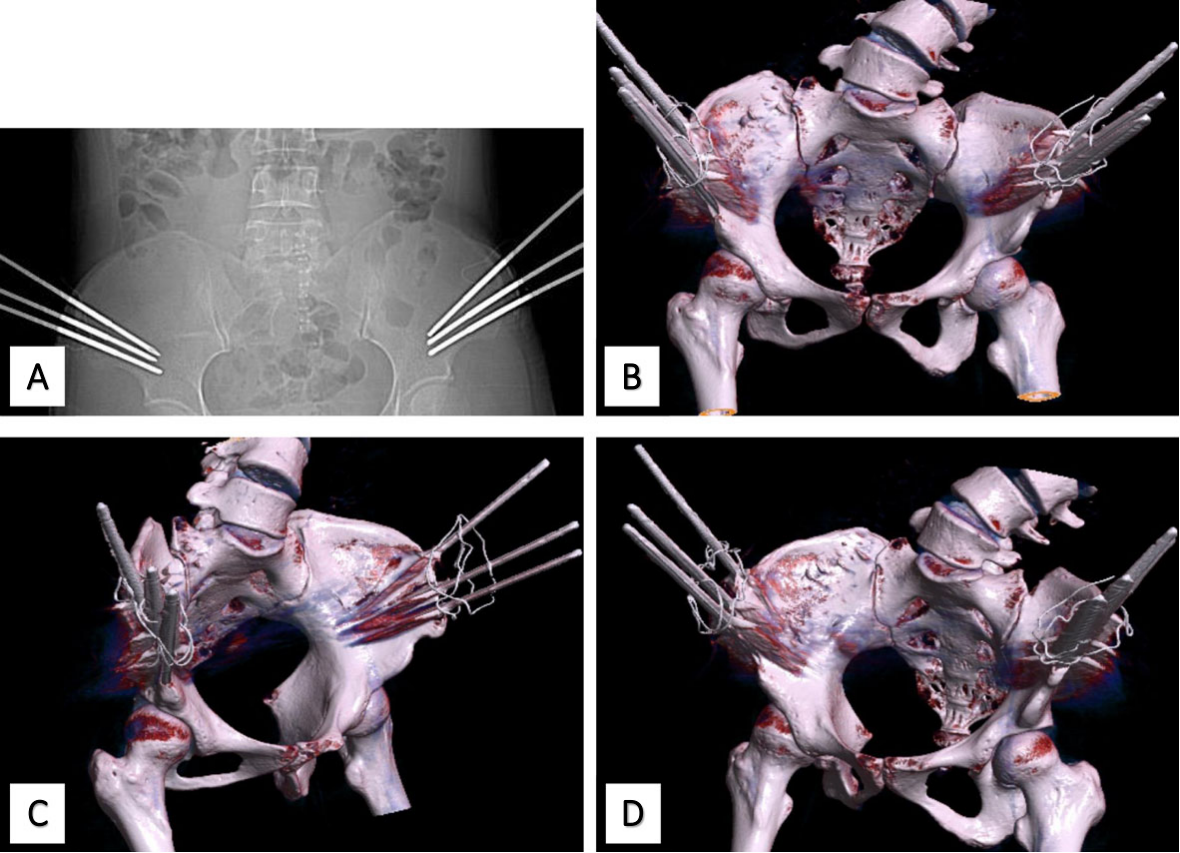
Photographs of bony pelvis with the pelvic pins. The anteroposterior digital radiographs (a), and the CT scan (b-d)
